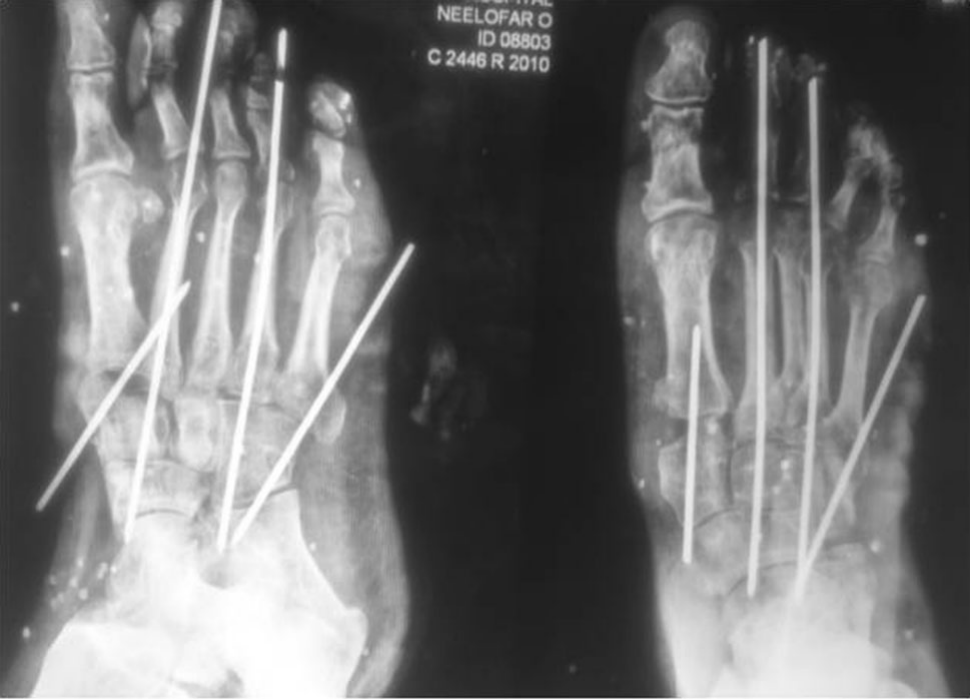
Oblique and AP radiograph of foot after open reduction and K wire fixation
